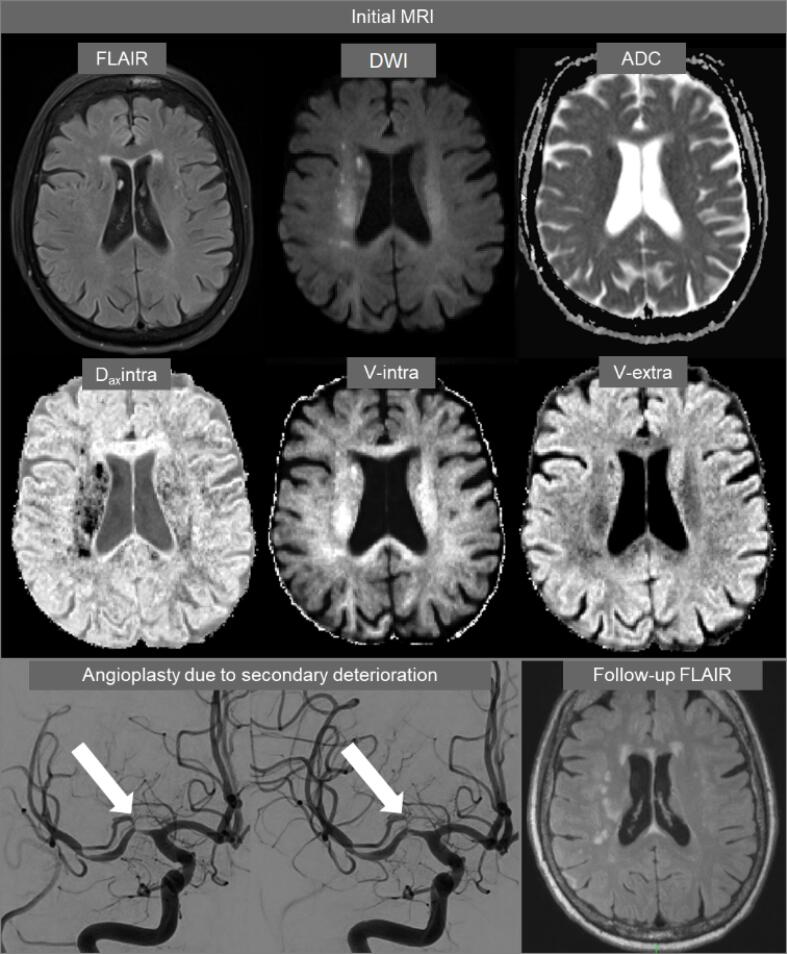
A 78-year-old woman presented with fluctuating paresis of the left arm and dysarthria due to high-grade stenosis of the right middle cerebral artery since 11 h. FLAIR showed no signal alterations, ADC revealed subtle ischemic changes in the deep border zone between perforating MCA branches and deep medullary arteries. A DWI (averaged over b ≥ 1800) shows already clear signal alterations, and DMI reveals a clear reduction in intra-axonal diffusivity (D_ax_intra), an increase in V-intra and concurrent reduction in the volume of V-extra. Following clinical deterioration after the MRI scan, the patient was treated with angioplasty. Note that the extent of ischemic changes on FLAIR follow-up is more consistent with the axial intraaxonal diffusivity map than ADC is.
